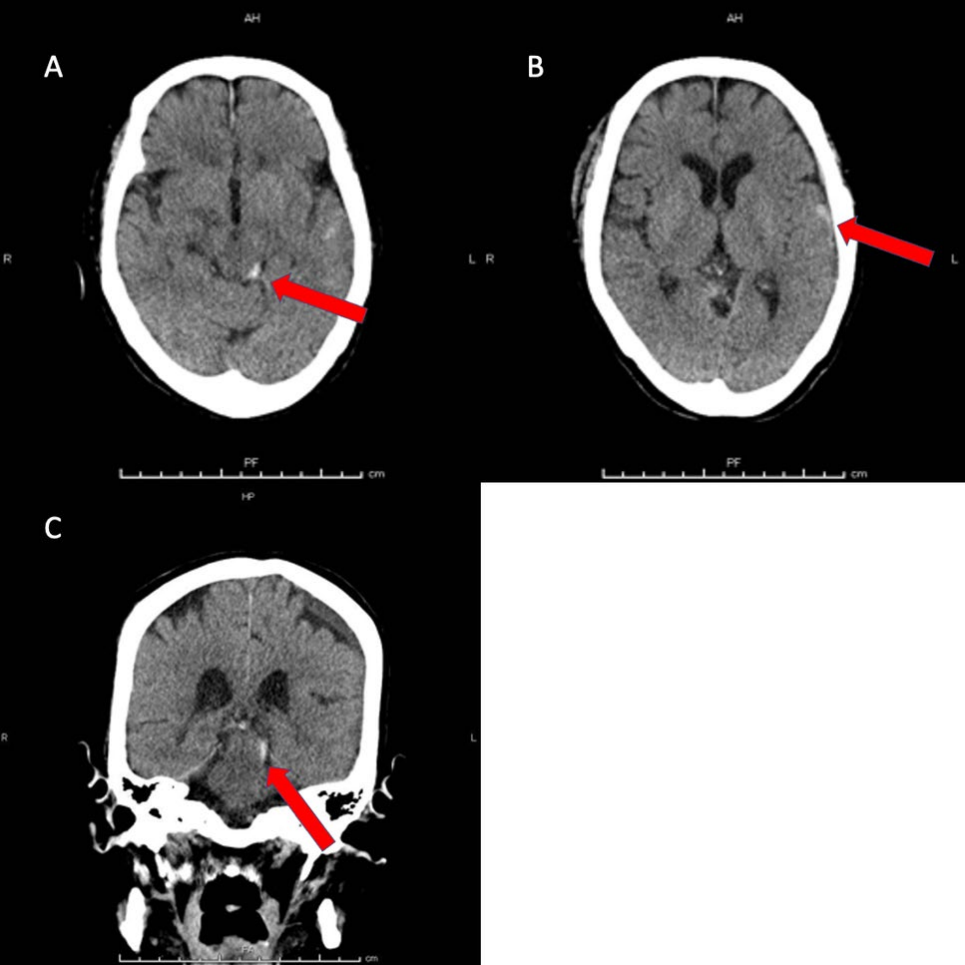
Axial computed tomography (CT) shows subarachnoid hemorrhage (SAH) around the midbrain (A) and left temporal lobe (B). Coronal CT of the midbrain shows SAH and brain contusion (C). Red arrows show the respective lesions
